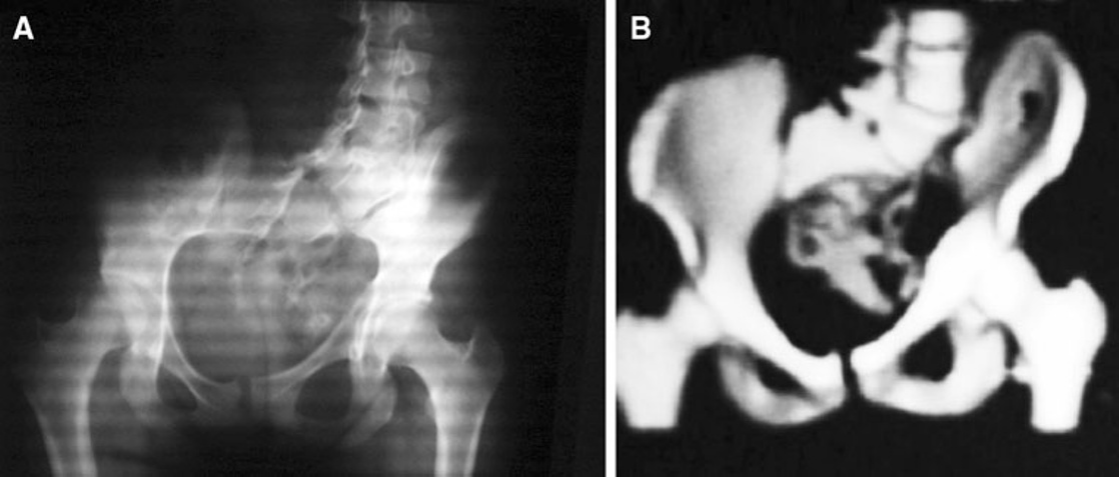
a A pelvic antero-posterior roentgenogram and a b, computed tomography scan of the pelvis with three-dimensional reconstruction revealed hemisacrum
